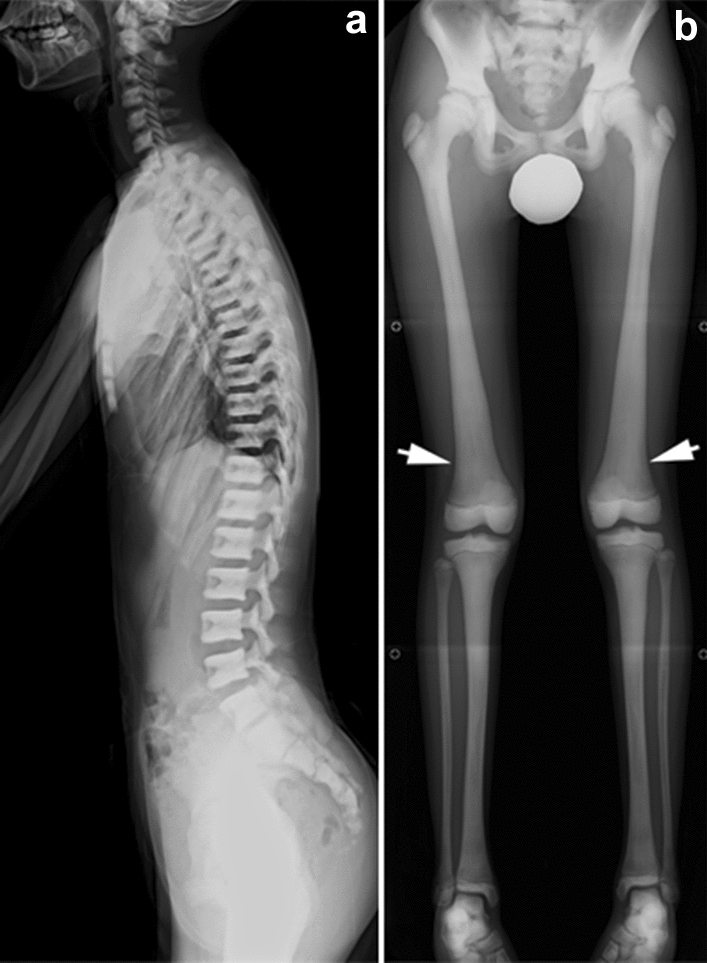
a, b A 14-year-old boy with adult type osteopetrosis. Radiographs show generalized increased bone density with poor corticomedullary differentiation and mild Erlenmeyer flask deformity of the long bones (arrows). Lateral view of the spine shows prominent superior and inferior endplate sclerosis, giving rise to a sandwich appearance of the vertebral bodies
